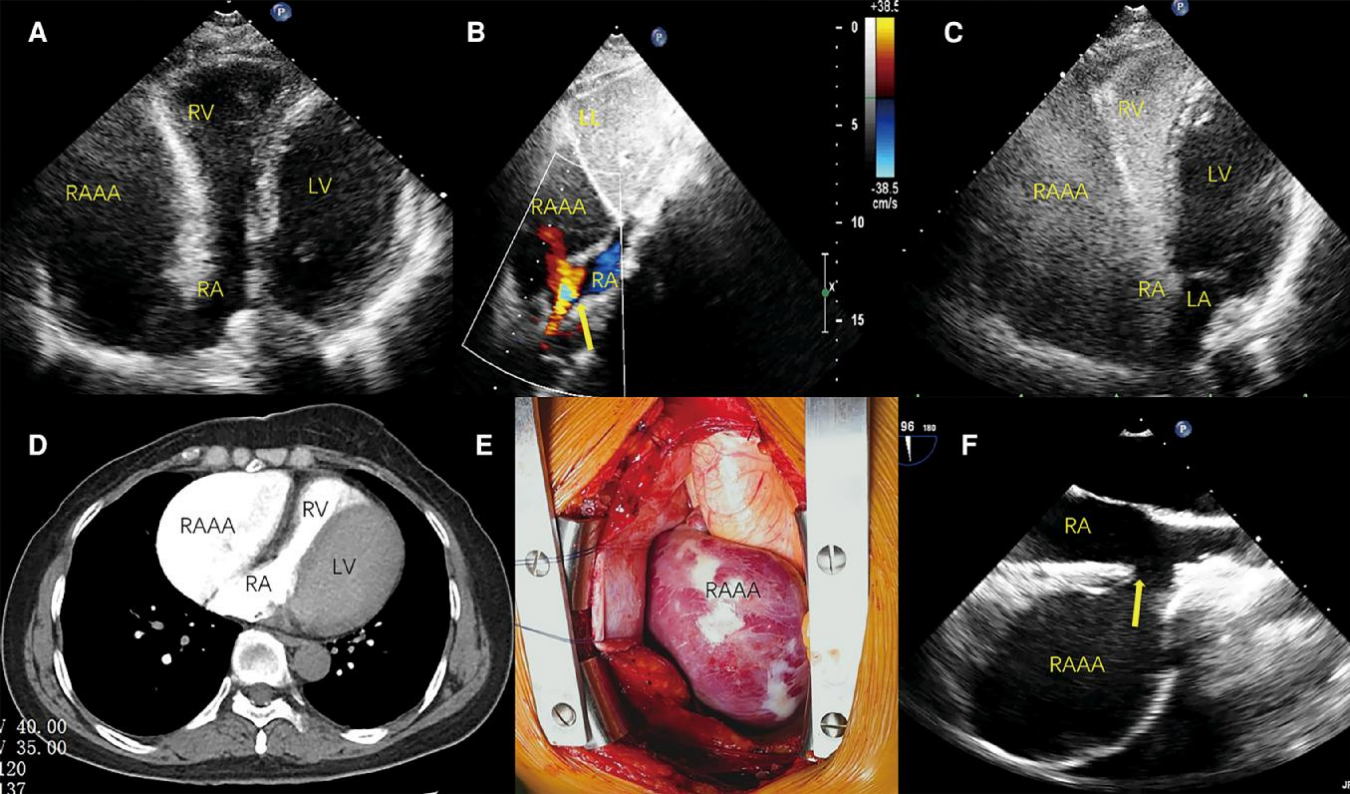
Right atrial appendage aneurysm (RAAA) on echocardiography. (*A*) The parasternal view shows RAAA pushing against the right heart. (*B*) In the subcostal view, the traffic between the RA and RAAA is observed (arrow). (*C*) The agitated saline microbubbles fill the right heart and RAAA. (*D*) Computed tomography angiography reveals no thrombus in the RAAA. (*E*) Intraoperative macroscopic shows the RAAA’s smooth surface. (*F*) Transoesophageal echocardiography displays RAAA’s smooth inner wall and the traffic between the RA and RAAA (arrow). RAAA, right atrial appendage aneurysm; RA, right atrial; RV, right ventricle; LV, left ventricle; LL, left lobe of liver; LA, left atrium.
